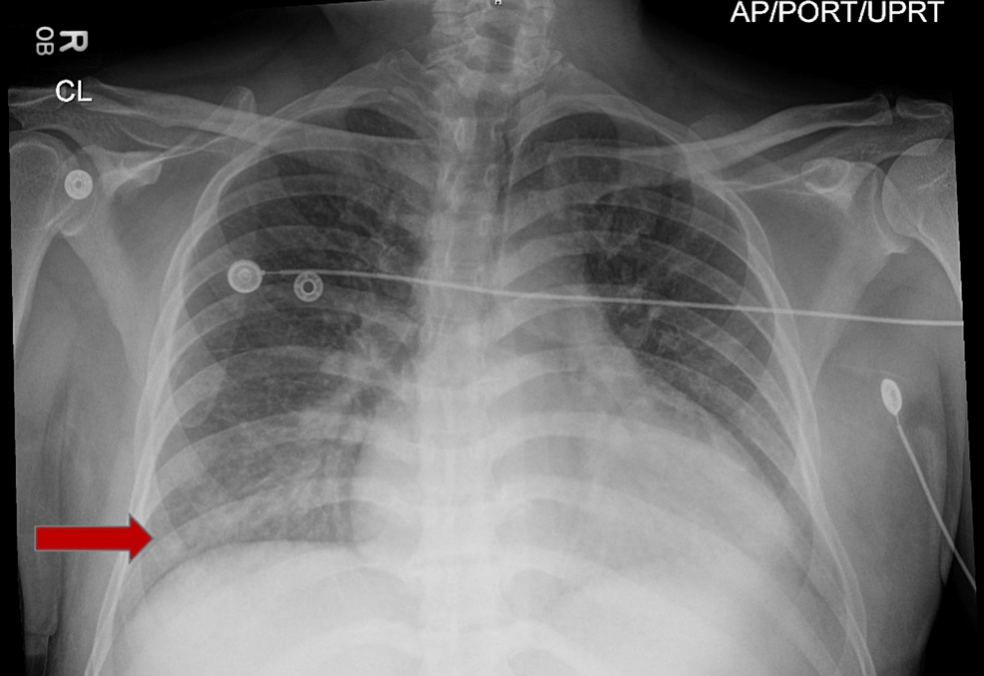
Chest X-ray showing pulmonary congestion and new ground-glass opacity in the left lower lung zone (red arrow)
